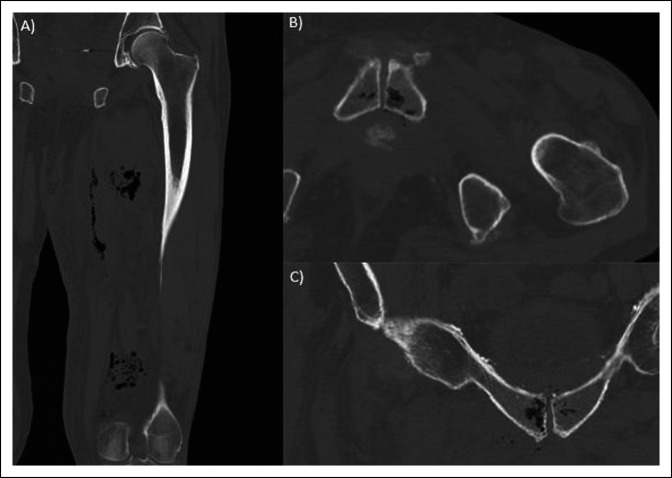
Computed tomography images from initial presentation. A, Coronal images of the thigh showing multiple foci of gas in the adductor compartment indicative of a necrotizing soft tissue infection. B, Axial and C, coronal images from pelvic CT showing intraosseous gas within the parasymphyseal bilateral superior pubic ramus.
